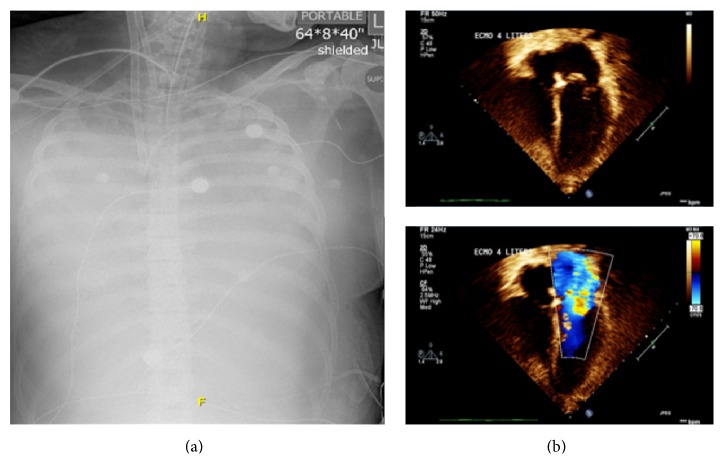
(a) Chest X-ray showing complete opacification of the thorax suspicion for complete lung consolidation secondary to edema. (b) Echocardiogram images showing flail mitral valve from a ruptured cord and severe mitral regurgitation.
